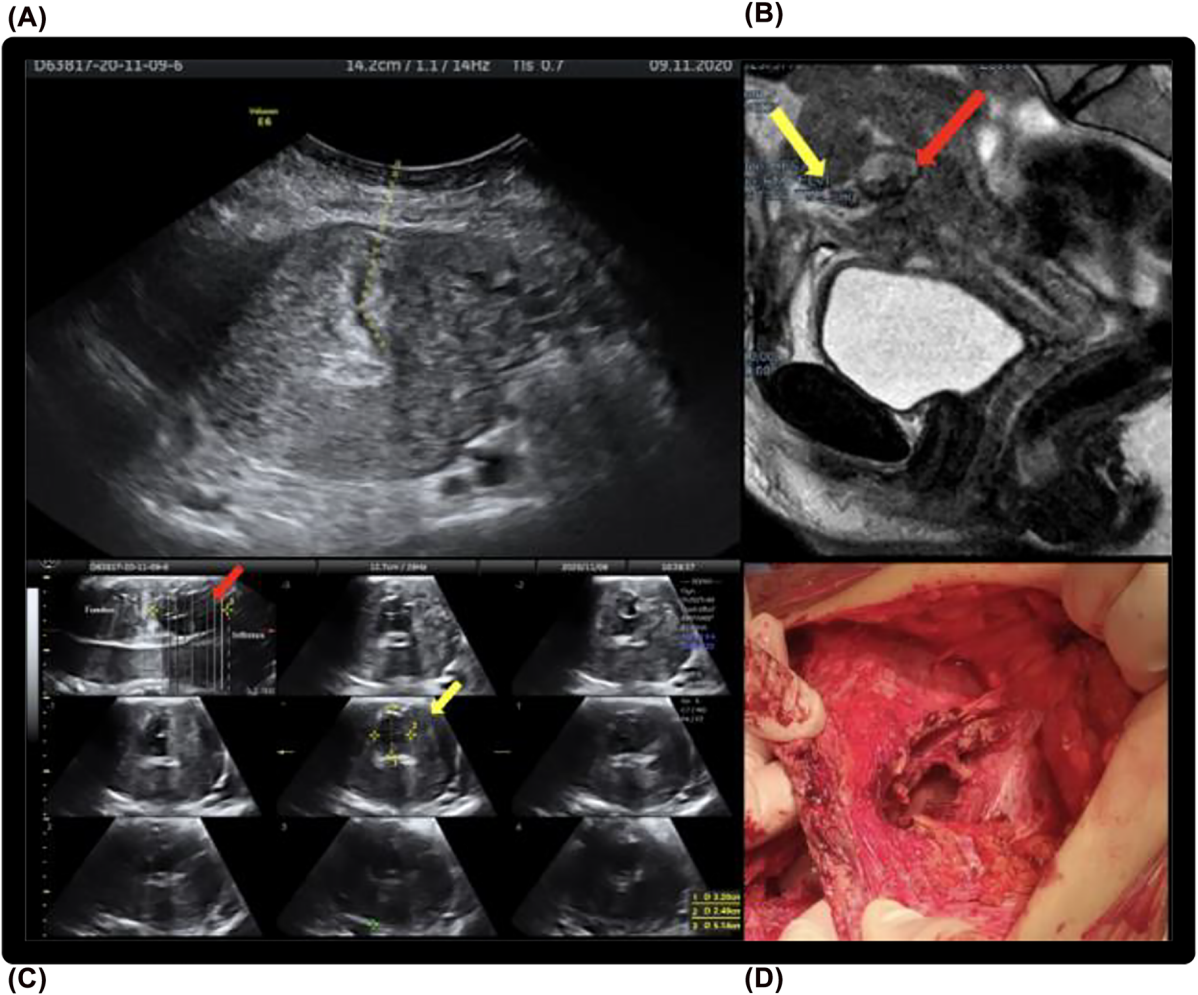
Case report 2. (A) Transverse ultrasound scan showing hypoechogenic fistulous tract between uterine cavity and anterior abdominal wall. (B) TUI 3D transabdominal US showing the major diameters of the fistulous tract: length equal to 5.14 cm in first sagittal plane image (red arrow); height of 3.20 cm and width of 2.49 cm in the axial plane of central image (yellow arrow). (C) Sagittal MRI scan of the patient showing the fistolous tract (yellow arrow) between uterine cavity and anterior abdominal wall; inferior to the fistula placental remnants are evident (red arrow). (D) Surgery: large fistula between anterior wall of uterus and the subcutaneous tissue of anterior abdominal wall at level of Pfannenstiel incision.
